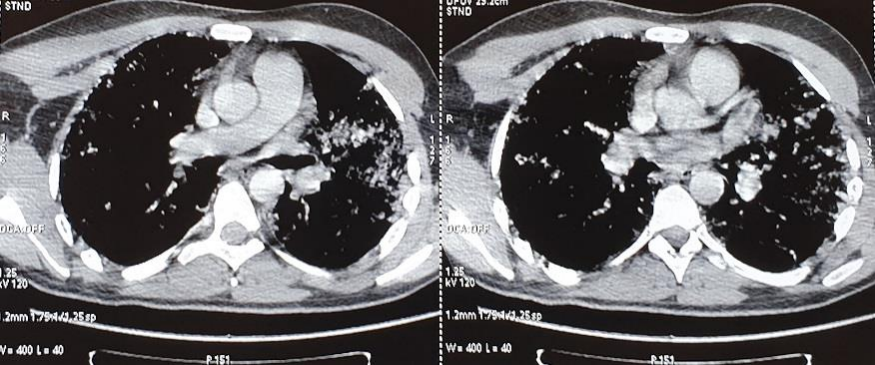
findings on computed tomography (CT) on admission showing no evidence of detectable pulmonary embolism
